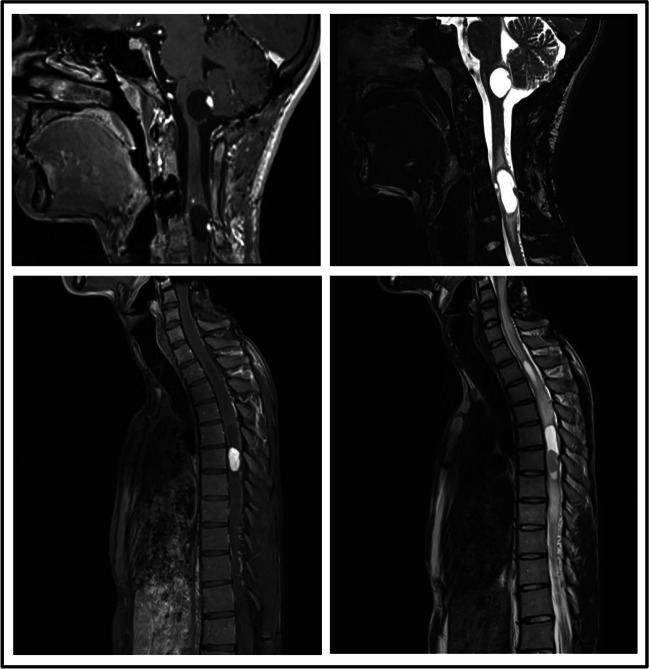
Contrast-enhanced T1 and T2 MRI sagittal cervical and dorsal images obtained from patient III-1, showing some of the hemangioblastomas with associated cyst that the patient presented
